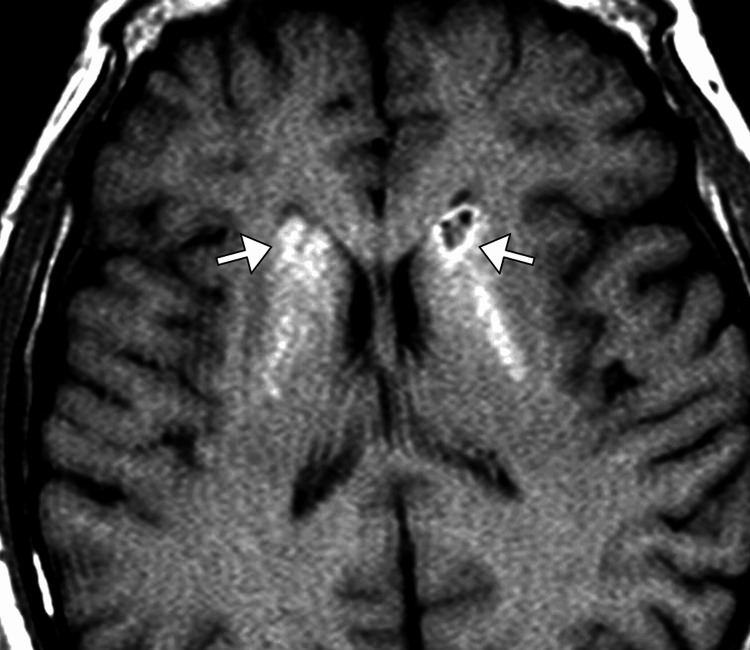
Axial T1-weighted MRI image of the brain showing bilateral dense calcification (arrows) of the basal ganglia.
